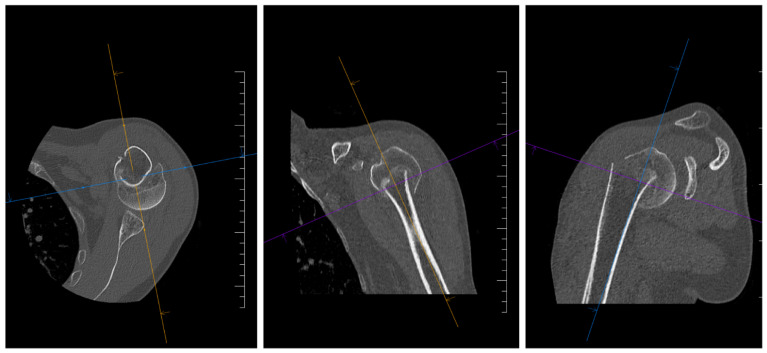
CT imaging of a three-part proximal humerus fracture during preoperative planning.
